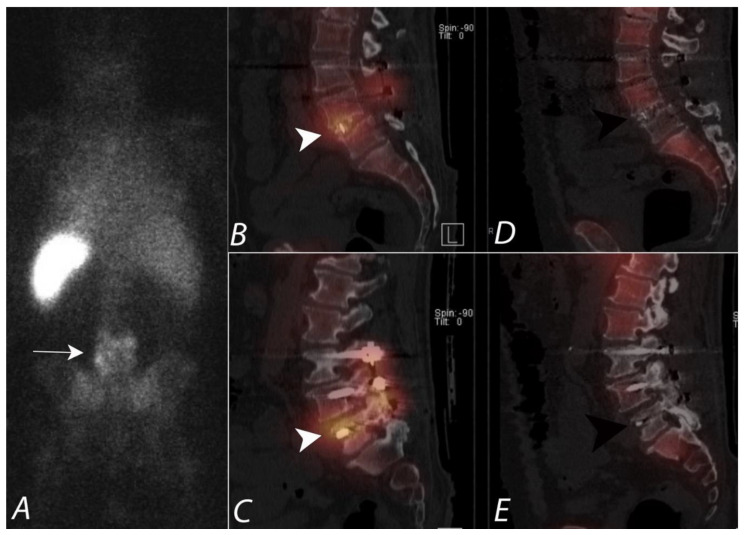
Seventy-two-year-old male with draining posterior lower lumbar wound one month after dorsal decompression and bilateral dorsal fixation from L3 to S1. Posterior planar image from an ^111^In leukocyte (WBC) scan (A) demonstrates heterogeneous uptake of tracer activity localizing to the lower lumbar region (white arrow). Sagittal SPECT/CT images of the ^111^In WBC scan (B,C) show increased uptake of labeled WBCs in the soft tissues, posterior elements, disc space, and vertebral bodies at L4-5. (white arrowheads). However, sagittal SPECT/CT images from ^99m^Tc sulfur colloid scan (D,E) demonstrate no focal uptake (black arrowheads) and is incongruent with the ^111^In WBC distribution of activity. This supports that a pyogenic infection is present involving the L4-5 vertebrae, posterior elements, and disc spaces. The absence of uptake on sulfur colloid confirms that the uptake of WBCs is not due to a granulation tissue due to mononuclear cell infiltration in chronic inflammation.
